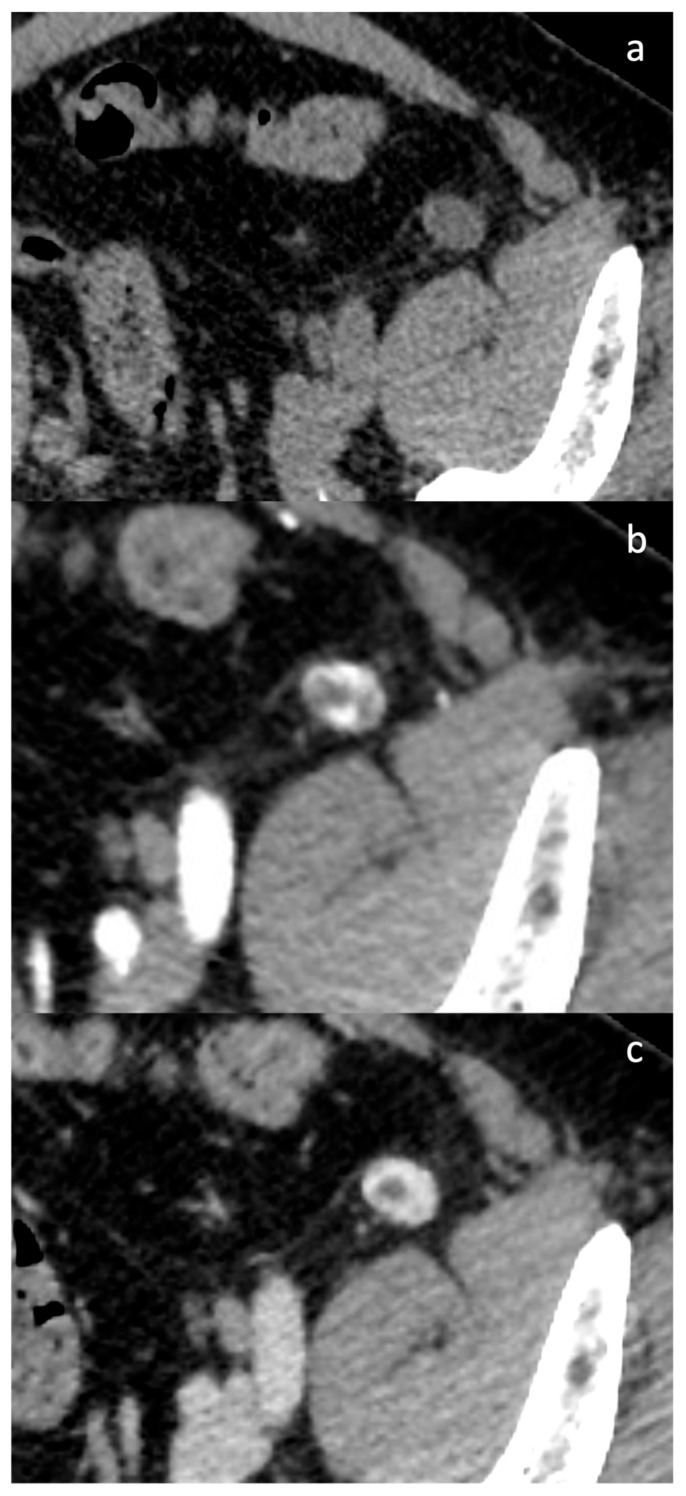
Axial CT scan details in the pre-contrast phase (a), arterial phase (b), and venous phase (c) with a demonstration of peripheral centripetal globular enhancement.
